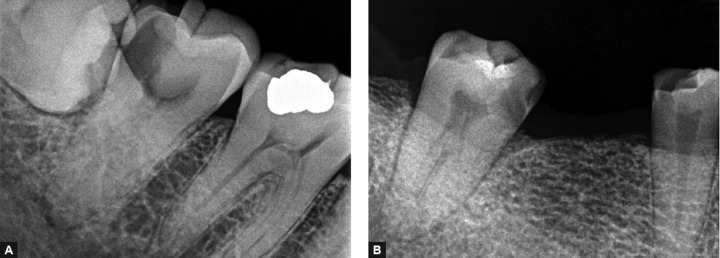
Radiographic appearance of a C-shaped canal configuration in mandibular second molar
